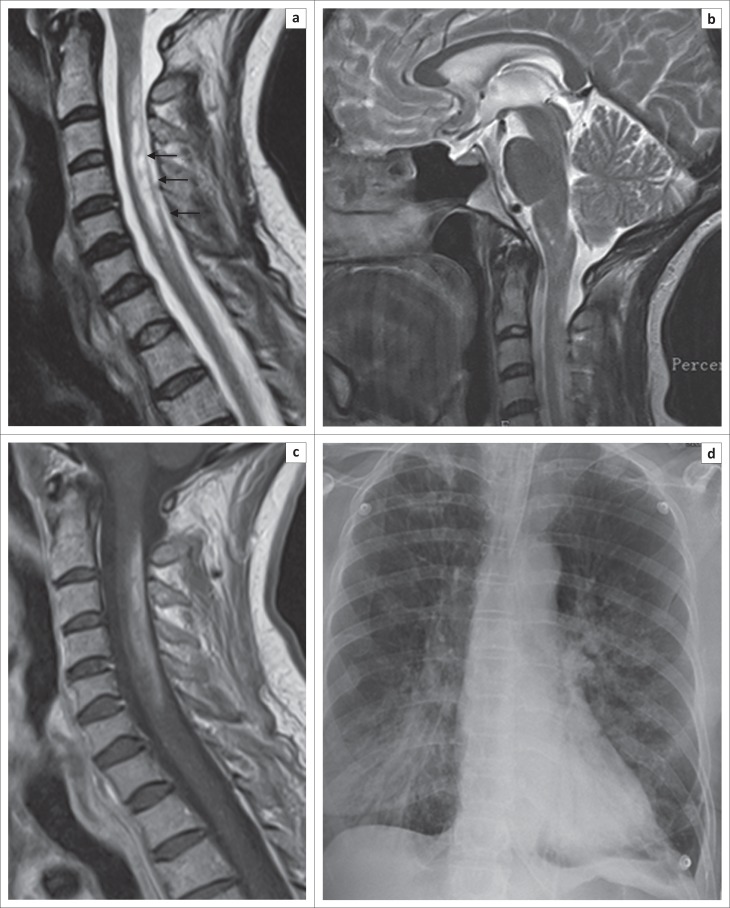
(a) and (b) are T2 sagittal images showing high intensity signal in the lower medulla and upper cervical cord; (c) is post-contrast T1 image showing enhancement of the lesion; (d) is a chest radiograph showing features consistent with tuberculosis.
